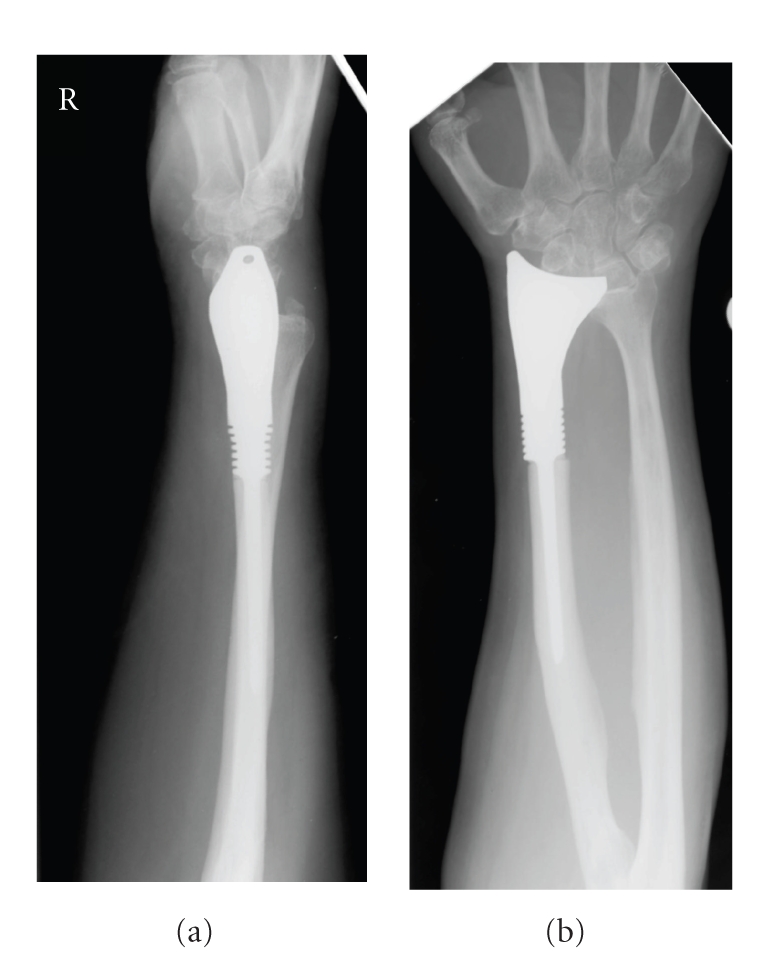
Radiographs of the distal forearm taken post-operatively at most recent follow-up, demonstrating the distal radial prosthesis and mild degenerative changes within the carpus.
